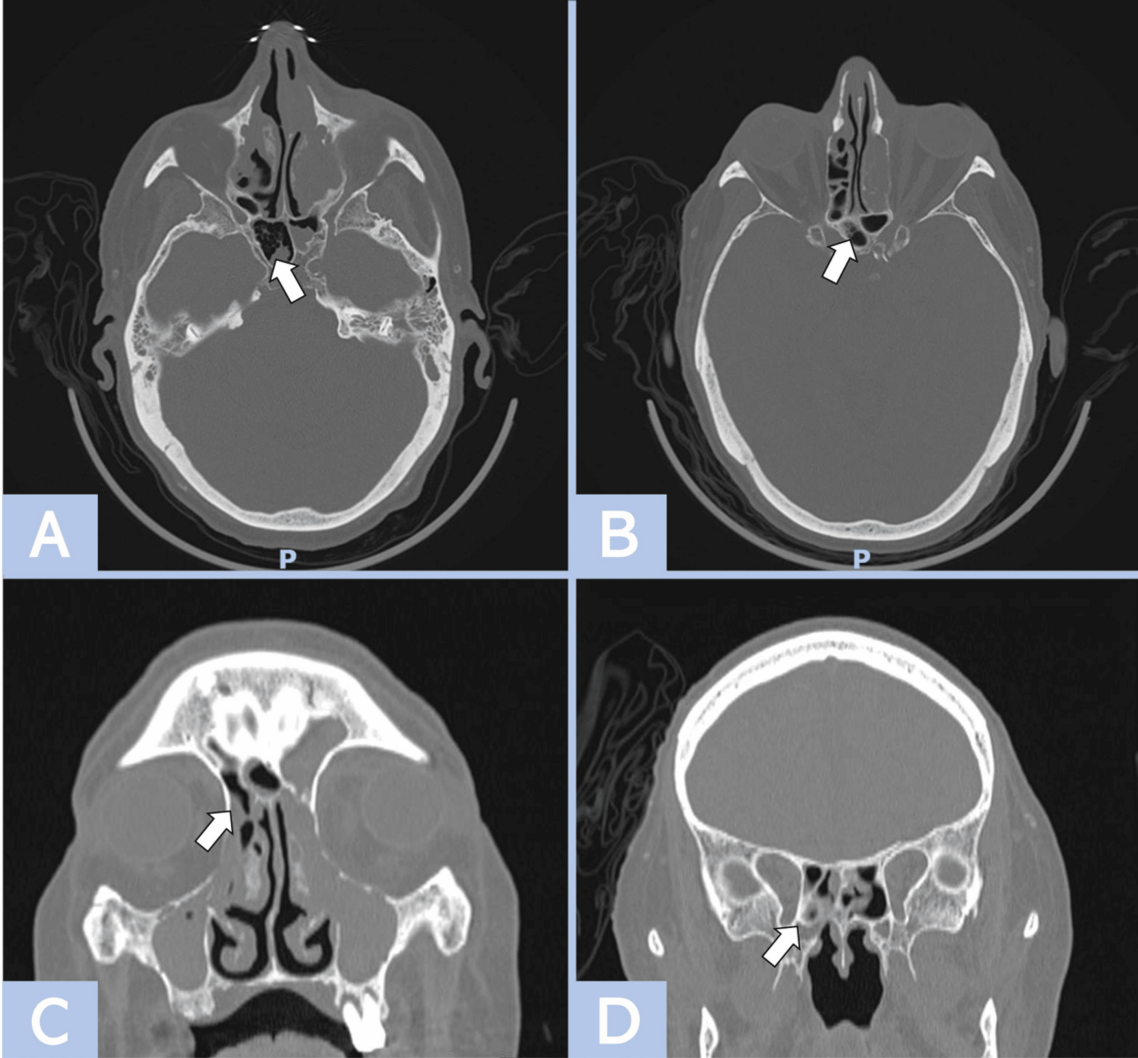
Plain skull CT revealing inflammatory changes. Plain skull CT, performed with axial (A-B) and coronal (C-D) cuts and viewed through a bone window, shows opacification of the maxillary, ethmoid, sphenoid, and frontal sinuses. The presence of air bubbles (marked with arrows) within the opacified areas suggests an acute process. Evidence of osteitis is observed in the ethmoid cells. Inflammatory changes are noted in the left orbit, including striation of the extraconal fat and eyelid edema, leading to proptosis. No fluid collections are identified. Extraocular muscles appear to be of similar thickness compared to the contralateral side.
